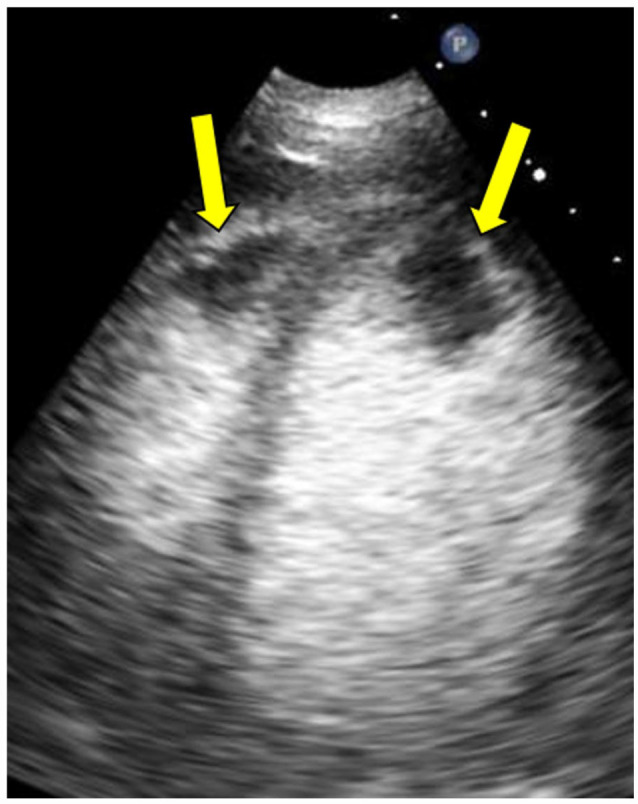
Transthoracic echocardiography with definity contrast, apical 4-chamber view. Arrows indicate large apical thrombi in the right and left ventricles.
